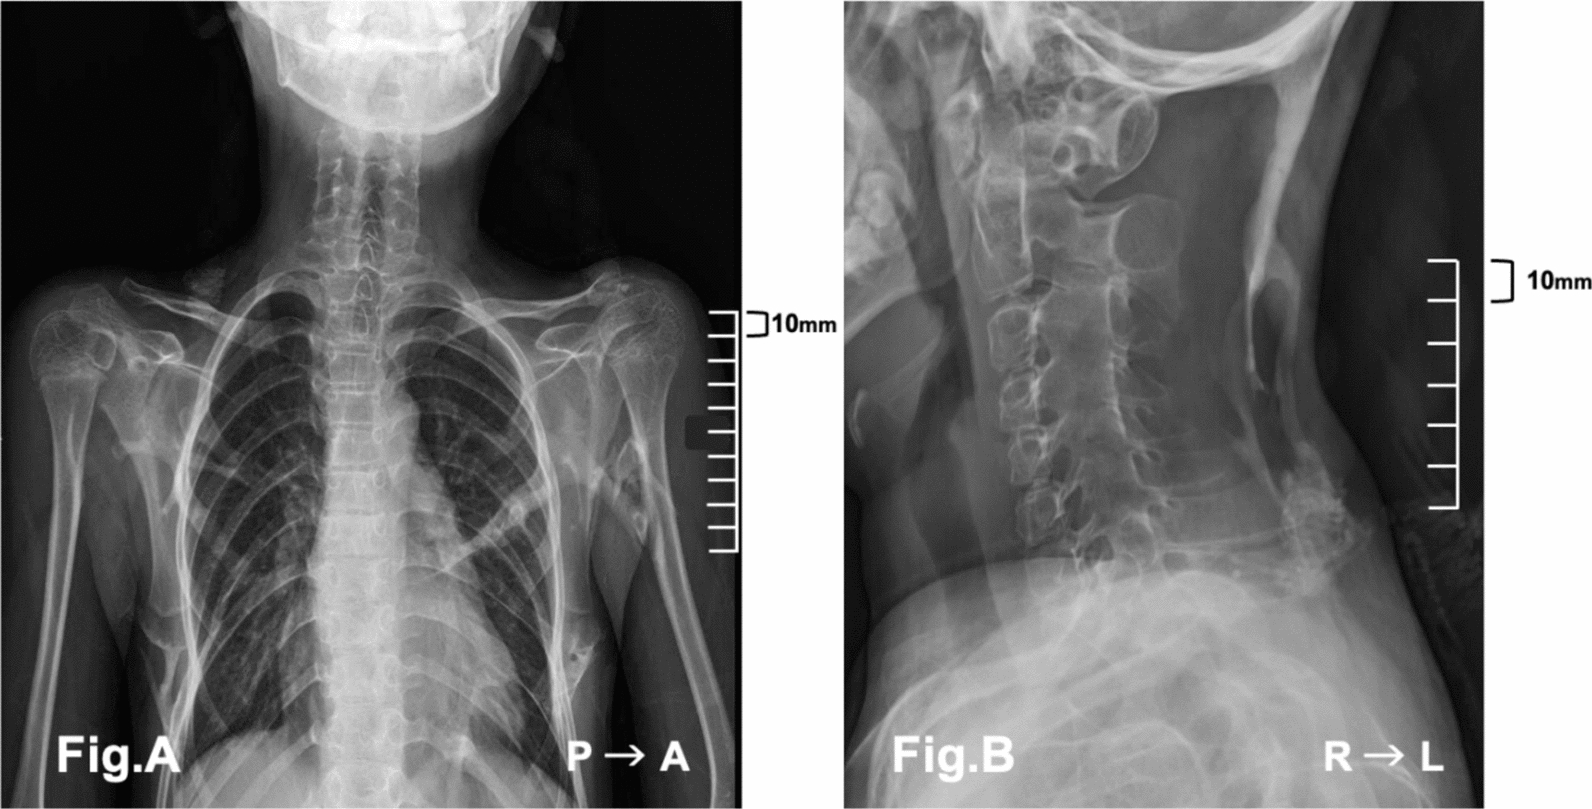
A Frontal chest radiograph. Preoperative imaging revealed cervical spine fusion and heterotopic ossification in the soft tissue adjacent to the inferior scapular border. However, no definitive thoracic cage deformity was evident at this stage. B Lateral cervical spine radiograph. Bony fusion was observed between the transverse and spinous processes of C2–C7. Subcutaneous heterotopic ossification was noted posterior to the C7 and T1 spinous processes. Additional ossification was observed between vertebral bodies, with irregularly shaped margins
